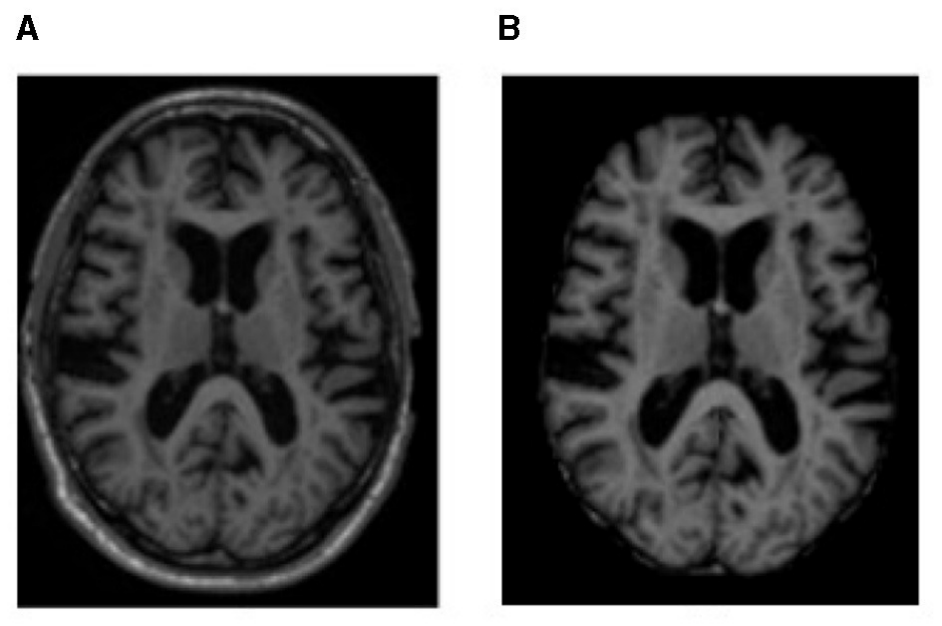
MRI sample representation from the dataset of (A) original MRI scan and (B) brain region image, i.e., an image after processing with BET to remove non-brain regions.
